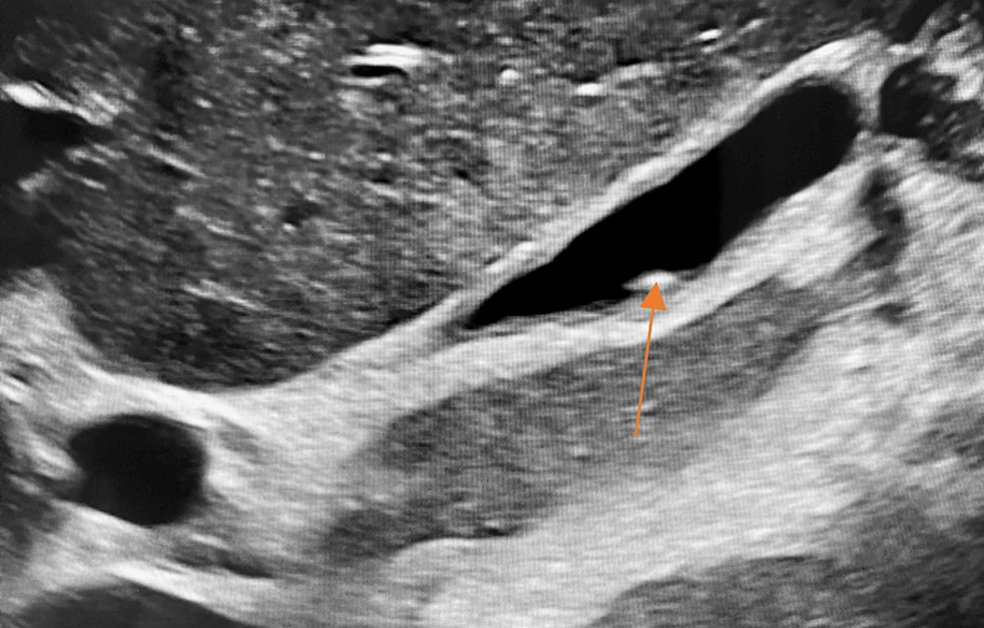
Abdominal ultrasound. Sagittal ultrasound view of the abdomen with a clear image of the gallbladder. A 6-mm stone can be appreciated within the gallbladder (orange arrow).
